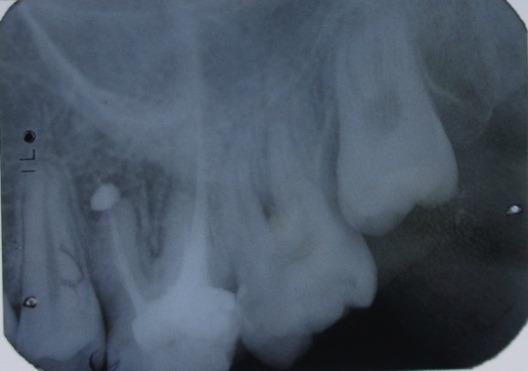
Periodontal space with periapical radiolucency at mesial and disto-vestibular root circumscribing apex -tooth 2.6
